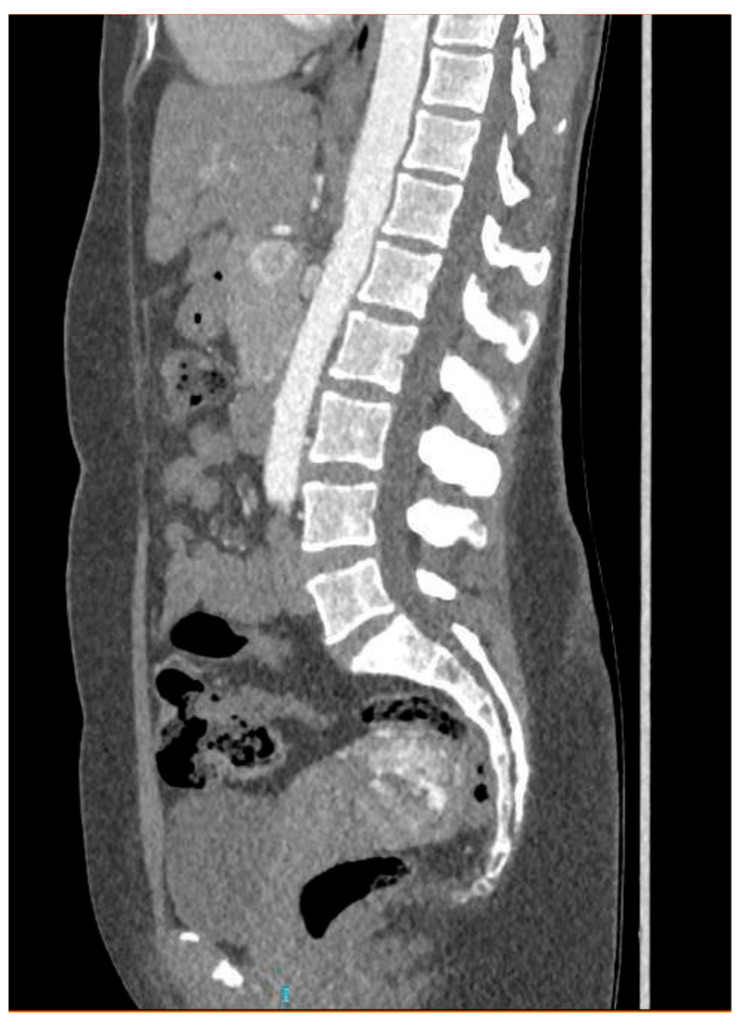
Coronal pelvic CT angiography.
